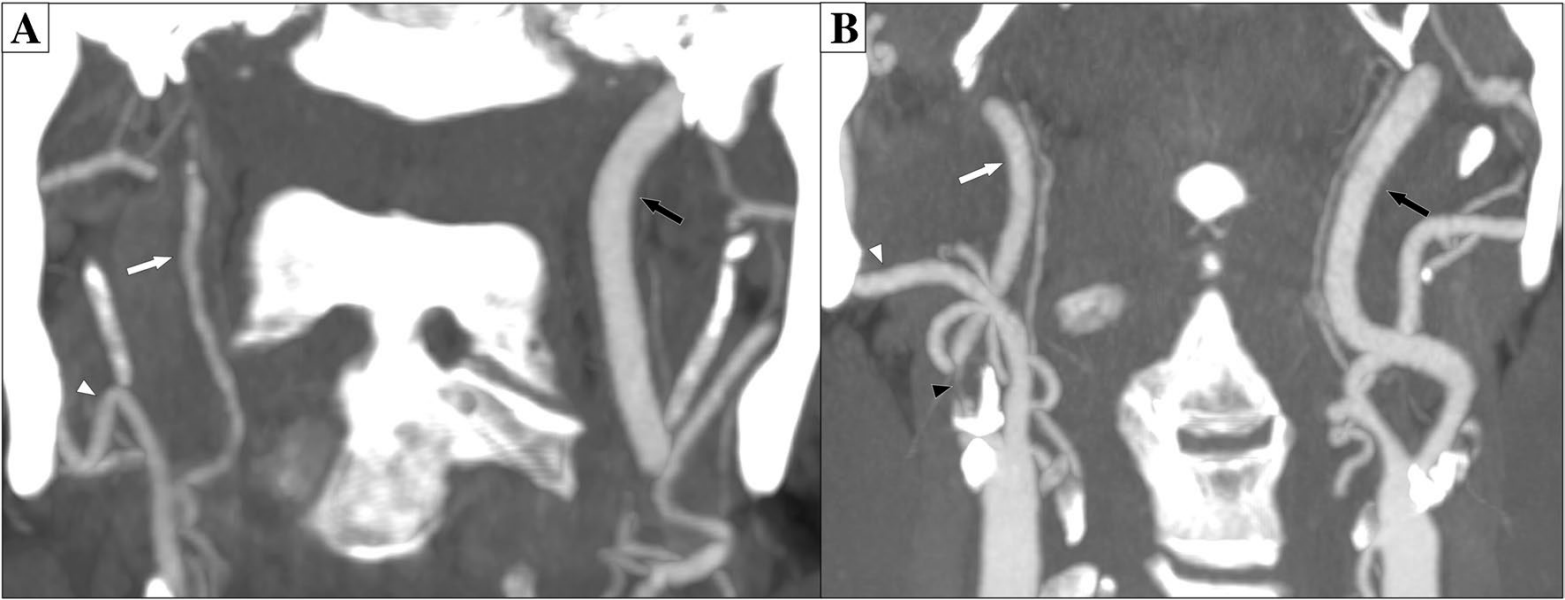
Two cases of right-sided near-occlusion on CTA. A Near-occlusion with full collapse. After severe stenosis (not in plane), distal ICA is threadlike (white arrow, 1.0 mm), clearly smaller than contralateral ICA (black arrow, 4.3 mm, ICA ratio 0.23) and smaller than ipsilateral ECA (white arrowhead, 2.1 mm). Full collapse by measurements and by appearance for both observers. B Near-occlusion without full collapse. After severe stenosis (black arrowhead), distal ICA is normal-appearing (white arrow, 2.4 mm), but smaller than contralateral ICA (black arrow, 4.3 mm, ICA ratio 0.56) and like ipsilateral ECA (white arrowhead, 3.0 mm)
